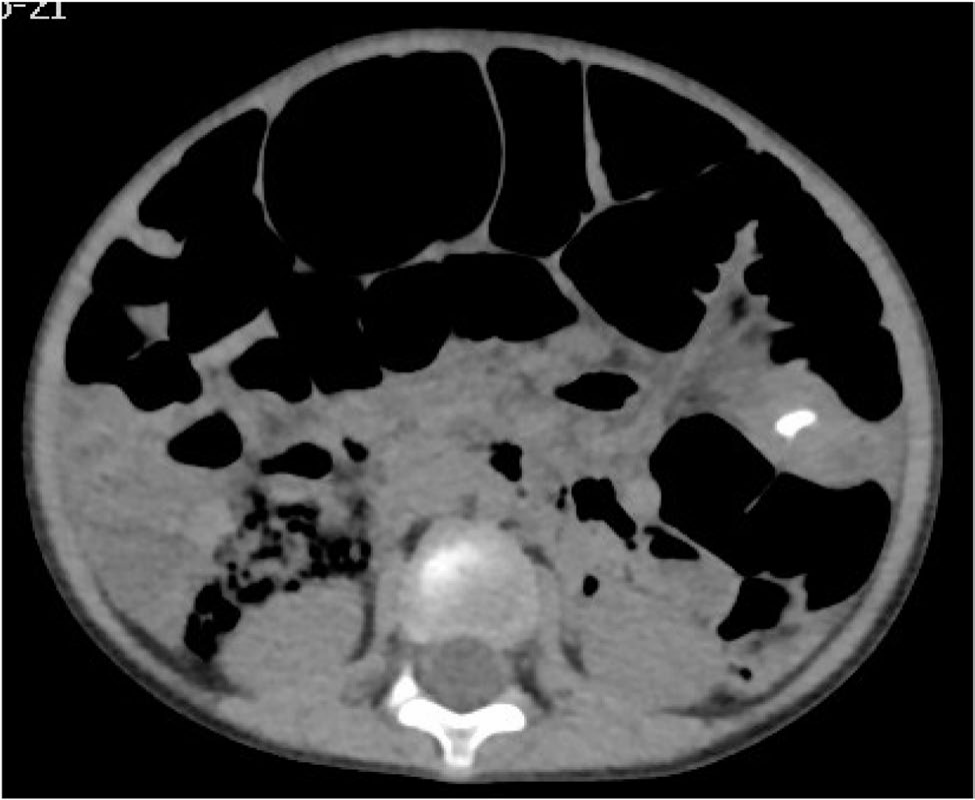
CT plain scan shows a high-density calcification shadow in the left mid-abdomen
